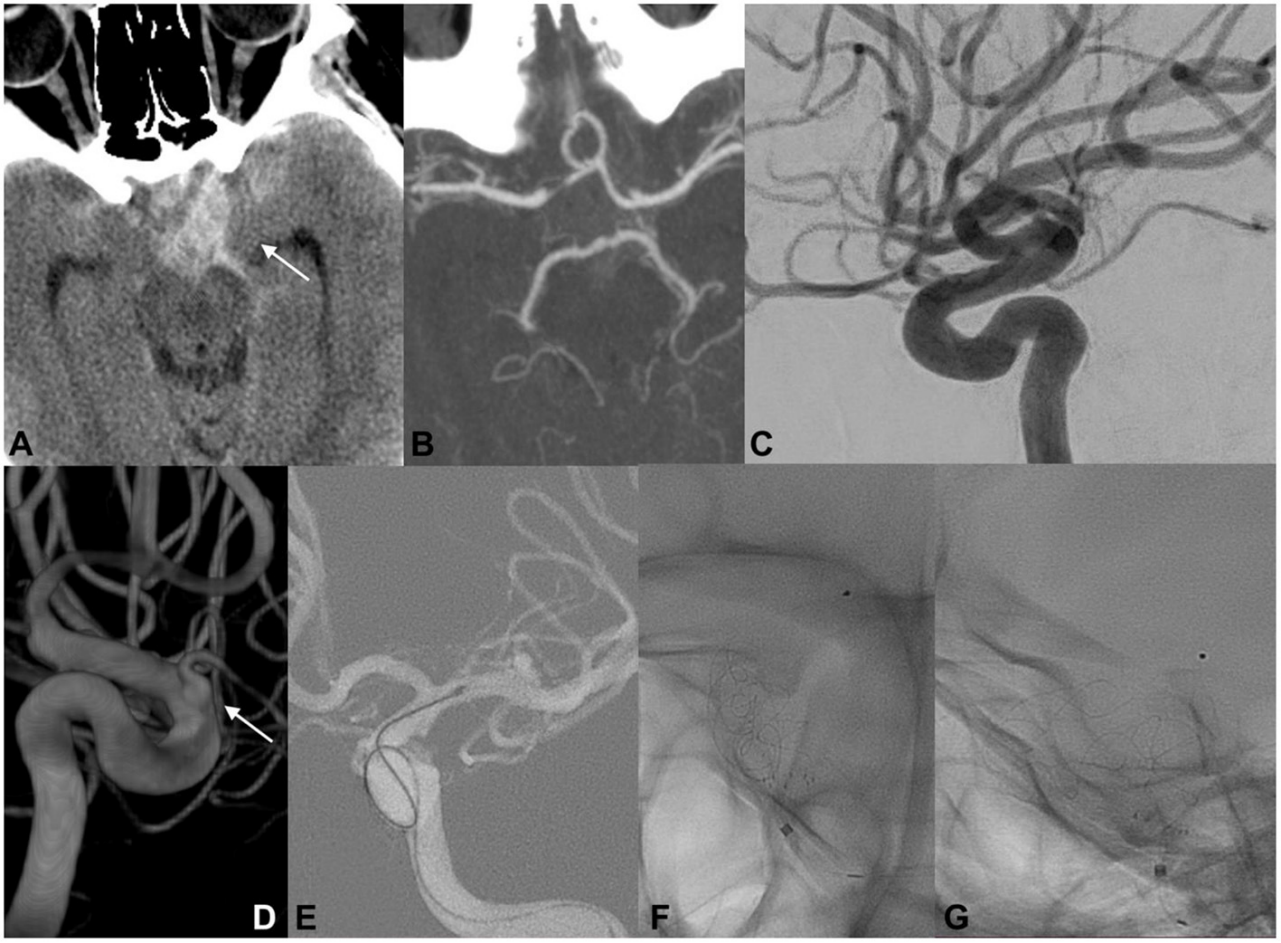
EVT of ruptured OA ID in a young woman. The initial radiological work-up indicated intracranial hemorrhage, suggesting the rupture of an intracranial aneurysm (A; white arrow). Cranial CTA and initial DSA did not reveal any cerebrovascular pathologies that could be associated with the hemorrhage (B,C). Three-dimensional rotational angiography demonstrated the presence of an ID at the level of the orifice of the left ophthalmic artery (D; white arrow). Endovascular FD deployment was performed with complete coverage of the OA infundibular orifice (E–G).
